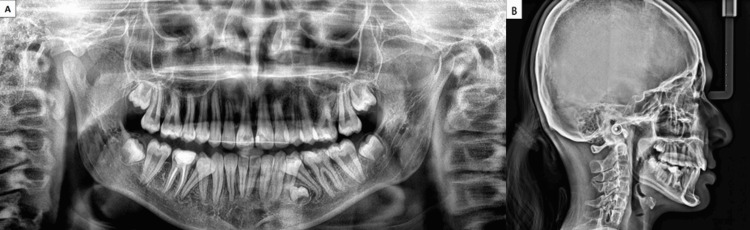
A. Postoperative orthopantomogram. B. Postoperative lateral cephalogram.
